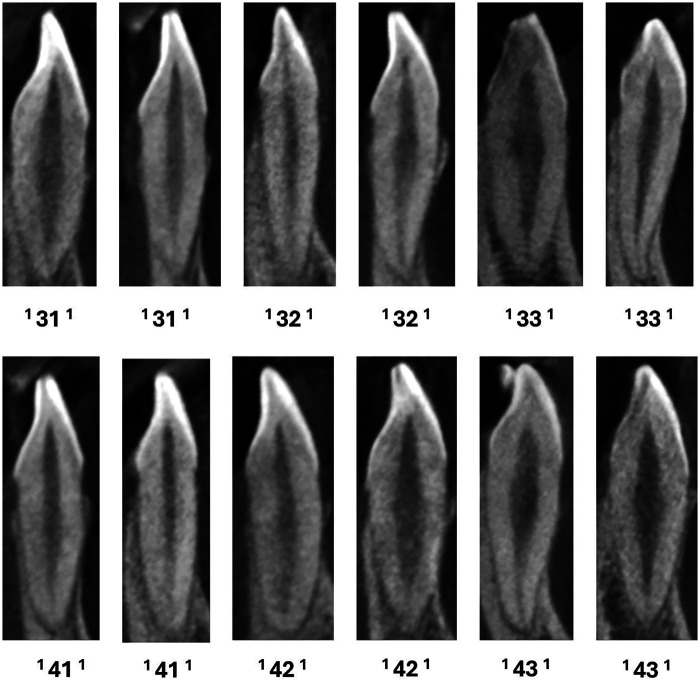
CBCT sagittal view of mandibular anteriors with a single canal.
